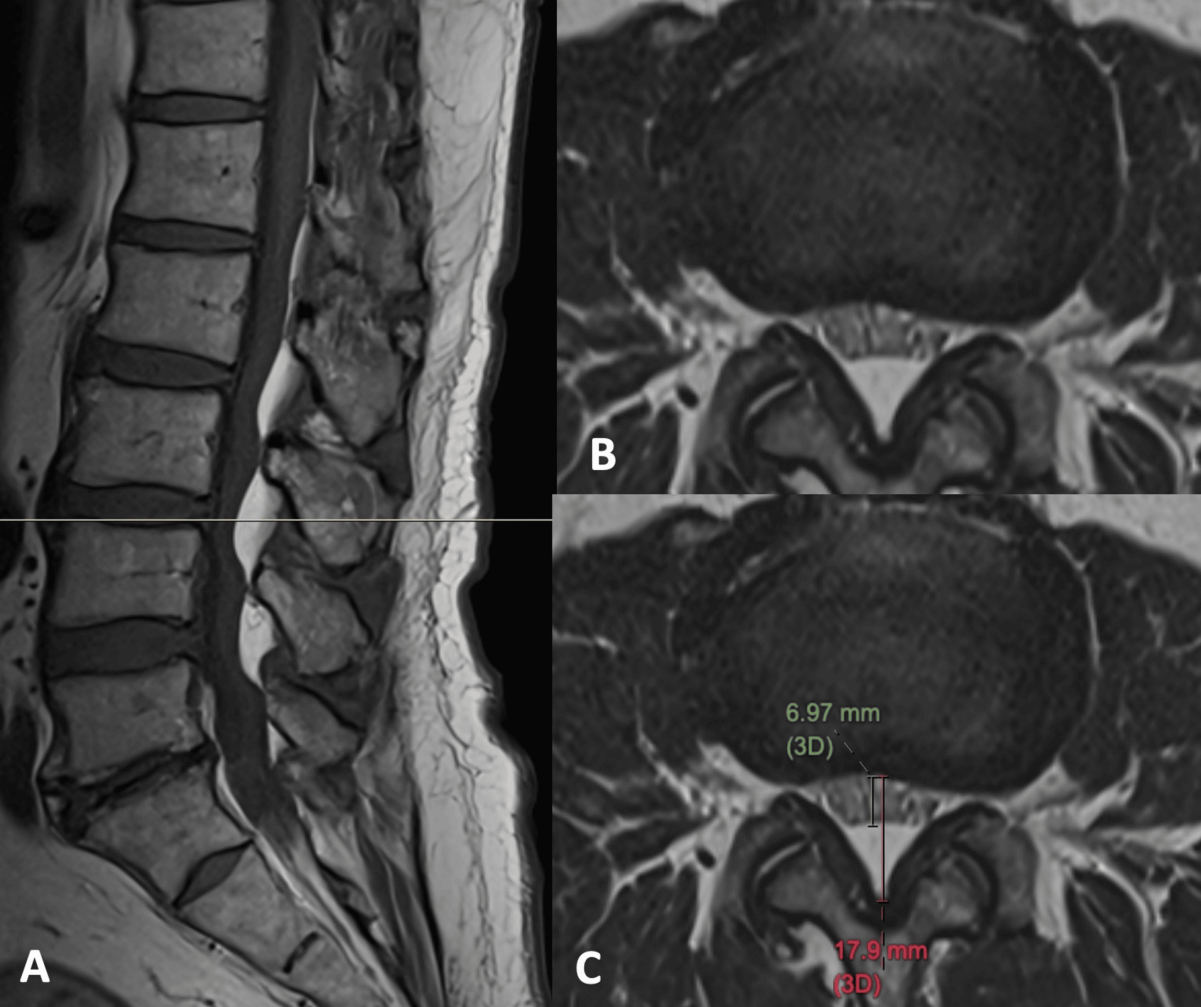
MRI of the lumbosacral spine of a 70-year-old man with a history of increasing low back pain for years with also radiation to the left leg. (A) Sagittal T1-weighted image shows increased epidural fat from the L2/3 to L4/5 levels. Lumbar spondylosis with marginal osteophytes and disc herniation are evident. (B, C) Axial T2-weighted images at the L3/4 level, near the superior endplate of L4, show an asymmetrical posterior disc herniation, which is more prominent on the left paracentral region. The dural sac is in polygonal shape with mild crowding of the cauda equina (dura/fat ratio = 0.64, fat% = 61%), moderate SEL. MRI: magnetic resonance imaging; SEL: spinal epidural lipomatosis.
